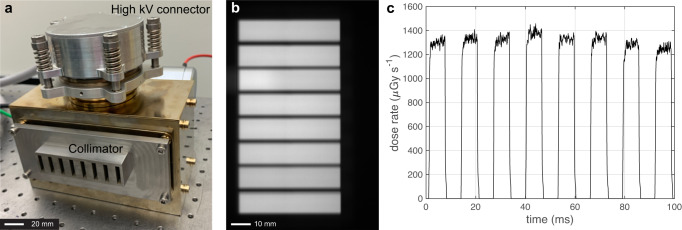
Photograph and characterizations of the carbon nanotube (CNT) field emission X-ray source array. a The photograph of the CNT field emission X-ray source array with the external multisource collimator. b The radiation fields formed by the eight collimated beams were recorded at 200 mm away from the focal line using a flat panel detector. c The X-ray dose rate profiles were measured at the detector surface using a dose meter (Raysafe X2, Unfors Raysafe AB, Sweden), with the source operating at 90 kVp, 15 mA anode current and 6.5 ms exposure per source with 0.3 mm Cu filtration.
